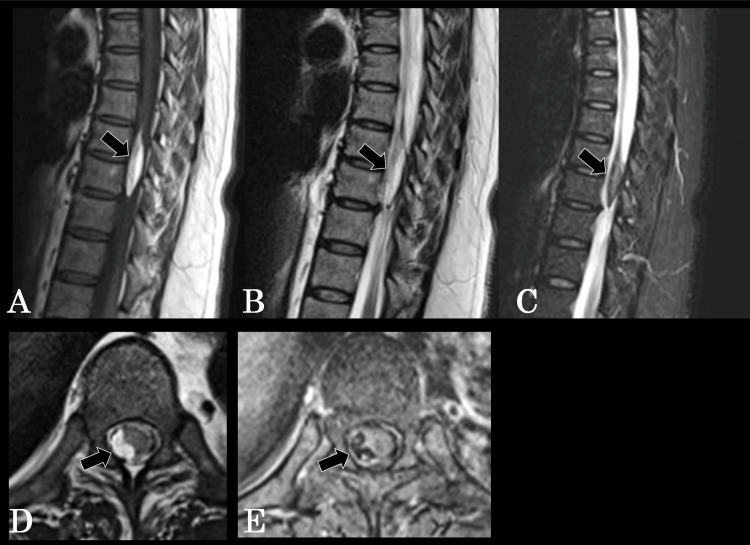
Thoracic spine MRI (A) T1-weighted image, (B) T2-weighted image, and (C) fat-suppressed image. (D) Axial T2-weighted image demonstrating a right-sided intradural extramedullary lipoma compressing the spinal cord leftward. The tumor area measures 39.51 mm², within a subarachnoid space of 114.58 mm², representing 34.5% occupancy of the available intradural space. (E) Axial fat-suppressed image showing complete signal suppression of the lesion, confirming its fat composition and depicting its relationship to the displaced spinal cord and adjacent nerve roots. MRI: magnetic resonance imaging
